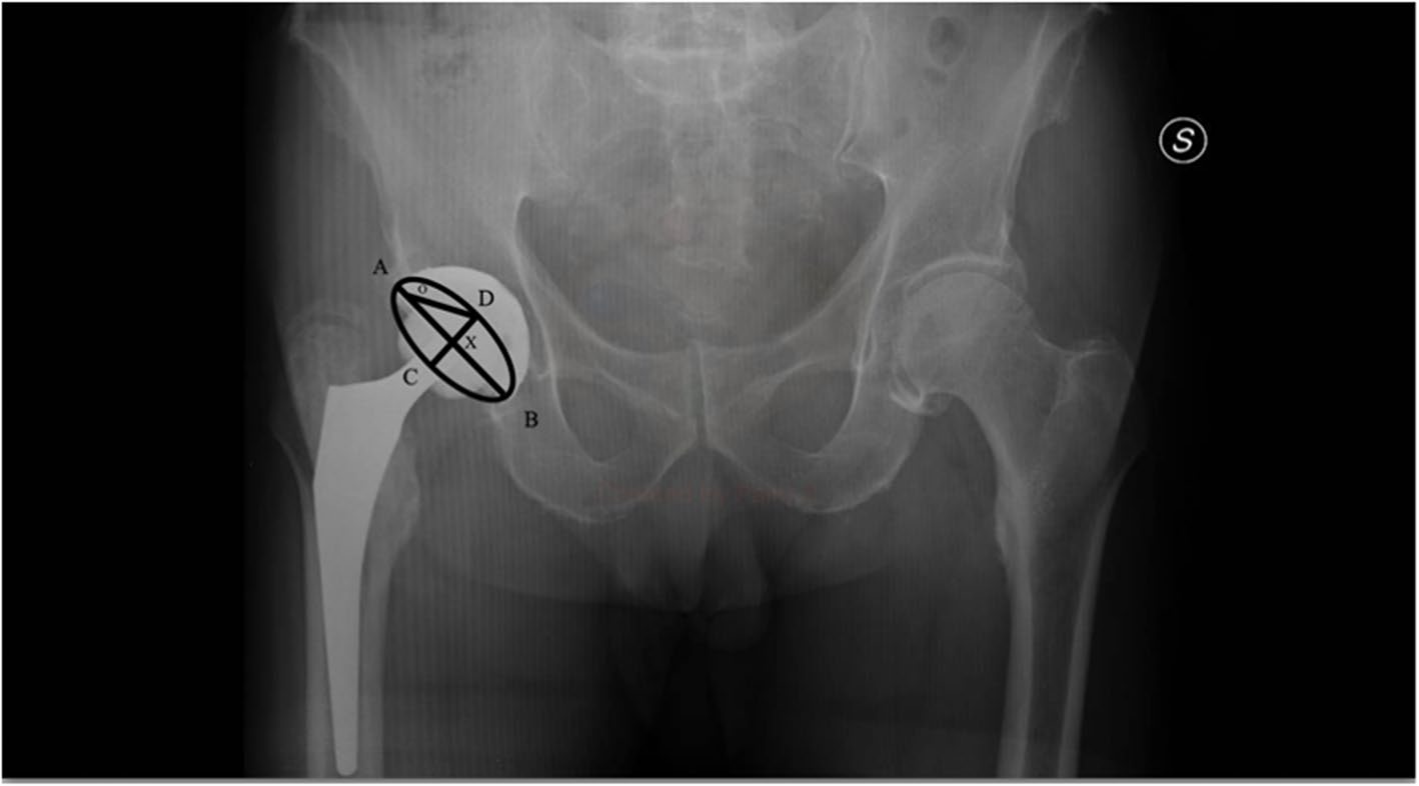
Acetabular cup ante-version. Calculation of acetabular cup anteversion on AP pelvis radiograph using method explained by V. Bachhal et al. AB = major axis of the ellipse, CD = minor axis of the ellipse represented acetabular component XOD = the calculated angle of anteversion
